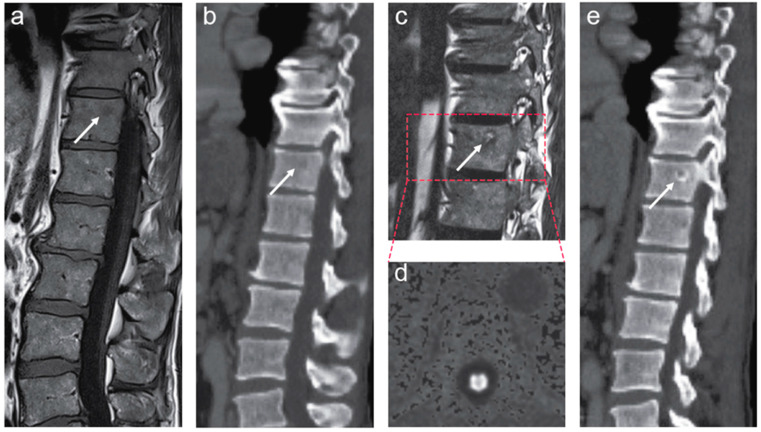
The left panel (a,b) shows pretreatment magnetic resonance imaging (MRI) and computed tomography (CT) scans of the thoracolumbar spine. Small, focal lesion in the thoracic spine is shown on MR ((a), white arrow) and CT ((b), white arrow) images. The right panel (c–e) shows follow-up MRI and CT performed after the start of combined androgen deprivation therapy. A sagittal T2-weighted fat-only Dixon image demonstrates an increased fat signal within the suspected lesion in the thoracic spine (c, white arrow). There was no evidence of tumor activity on the diffusion-weighted MRI (DW-MRI) (d). CT of the thoracolumbar spine showed subtle perilesional sclerosis ((e), white arrow). The findings (c–e) have appeared since the pretreatment MRI and CT scans and are presumably consistent with the treatment effect in solitary bone marrow metastasis.
